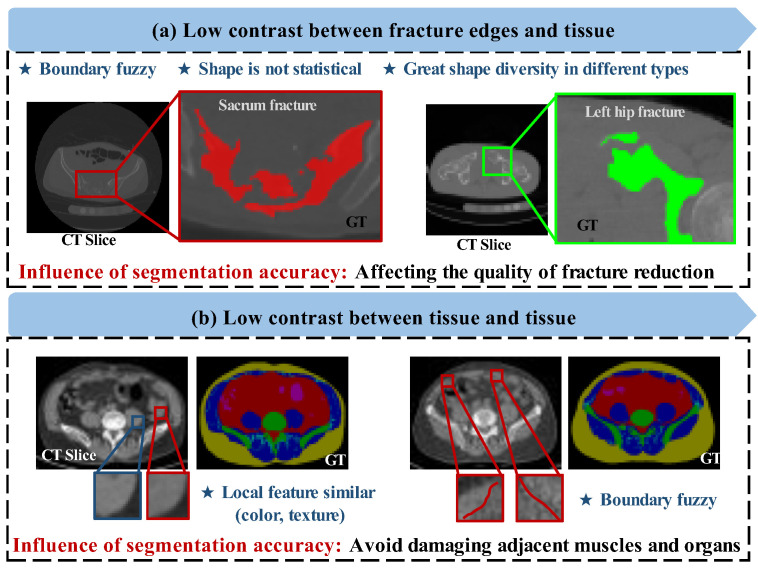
Some difficult samples of pelvic CT slices. (a) Low contrast between fracture edges and tissue. (b) Low contrast and local similarity between tissue and tissue. The boxes are the “interested region”, and the colors used are their corresponding GT marking color. The difficulties of segmentation are listed with asterisks.
